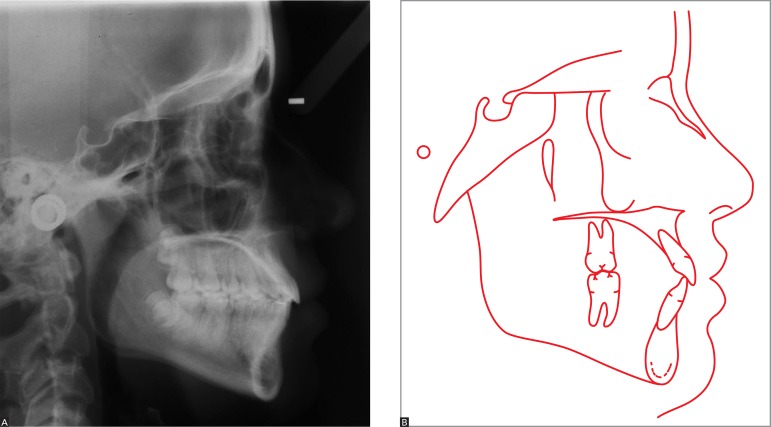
Final profile cephalometric radiograph (A) and cephalometric tracing
